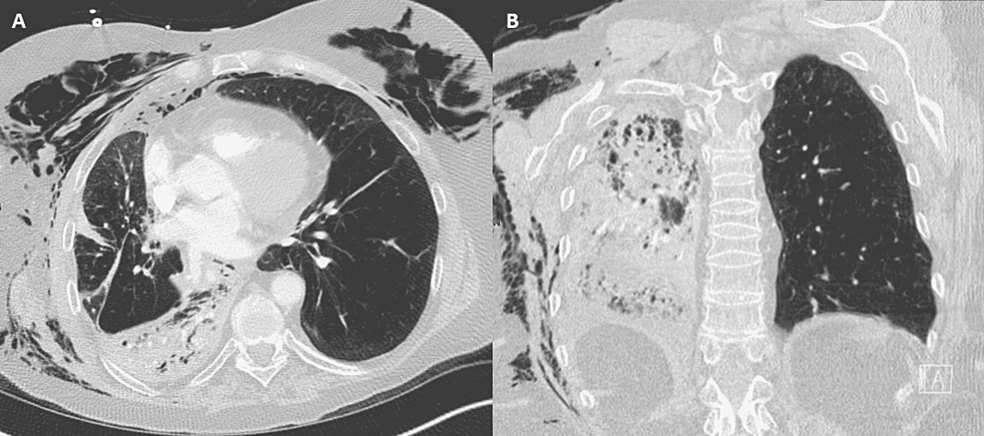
Axial (A) and coronal (B) chest CT images showing a dense right-lower lobar pneumonia. Extensive subcutaneous emphysema is also present. CT: computed tomography
